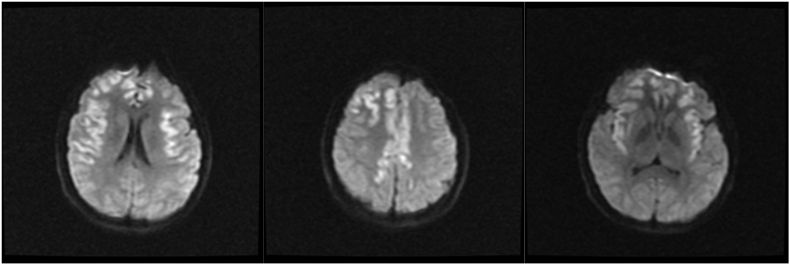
A, B, and 3C: Diffusion Weighted MRI showing signs of acute infarction in the temporal and frontal lobes.
